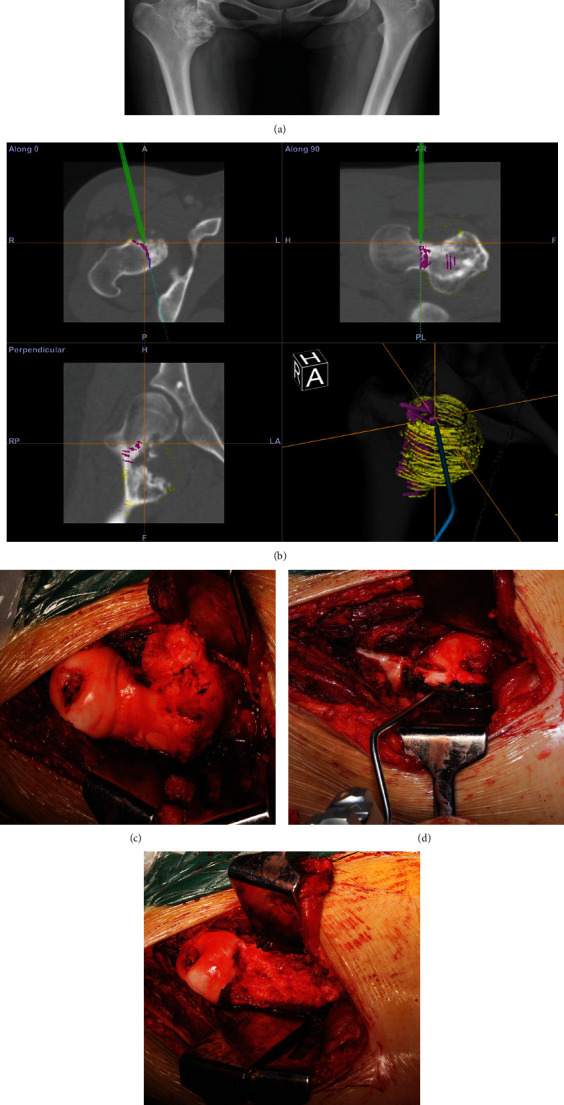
Preoperative planning and intraoperative pictures of patient 6. (a) Preoperative AP pelvic radiographs. (b) Computer screenshot during intraoperative navigation depicting axial CT image, coronal, and sagittal reconstructions. Preoperative plan for osteotomy marked in magenta. The green line depicts intraoperative position of navigation stylus. The yellow circles depict the pre-233 operative plan for resection margin. (c) Exposure via a posterolateral approach. (d) Osteotomy plane marked using a stylus. (e) Femoral neck following tumor resection.
